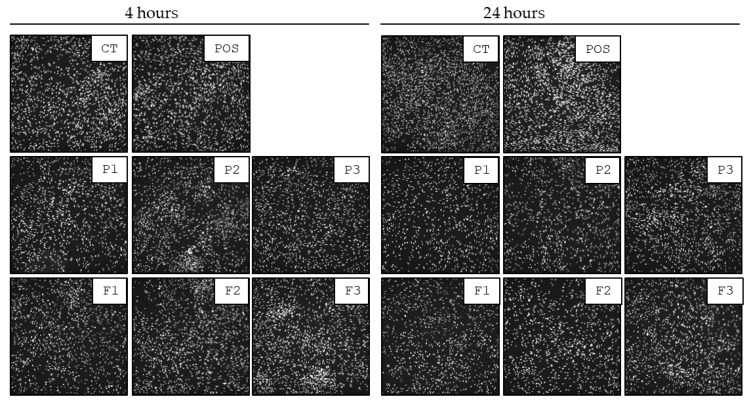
Representative microphotographs of cellular nuclei of human skin fibroblasts upon exposure to tested formulations without POS (P1–P3), containing POS (F1–F3) and untreated cells (CT) after 4 and 24 h. Scale ~500 µm.
